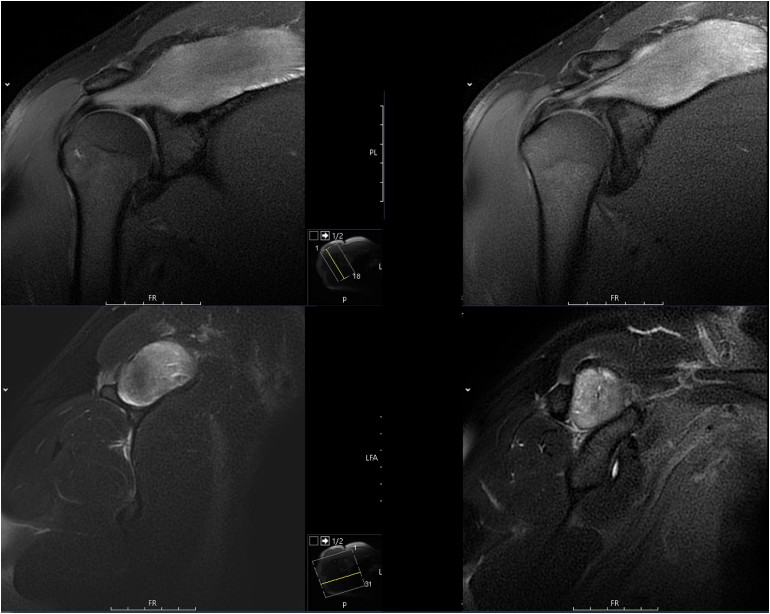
Magnetic resonance imaging of the right shoulder demonstrating increased signal and enlargement of the supraspinatus. Upper, proton density (PD) weighted sequence; lower, T2-weighted sequence.
